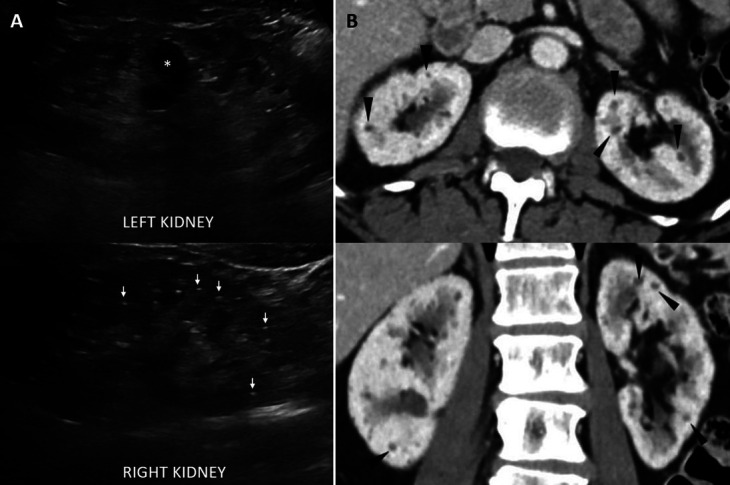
Radiologic appearance of lithium induced kidney cysts. (A) Kidney ultrasound images demonstrating multiple punctate echogenic foci and associated tiny echolucent areas (asterisk corresponds to a macrocyst and arrows point to a few representative microcysts). (B) Microcysts noted on CT imaging (arrowheads on a few representative lesions). CT, computed tomography.
